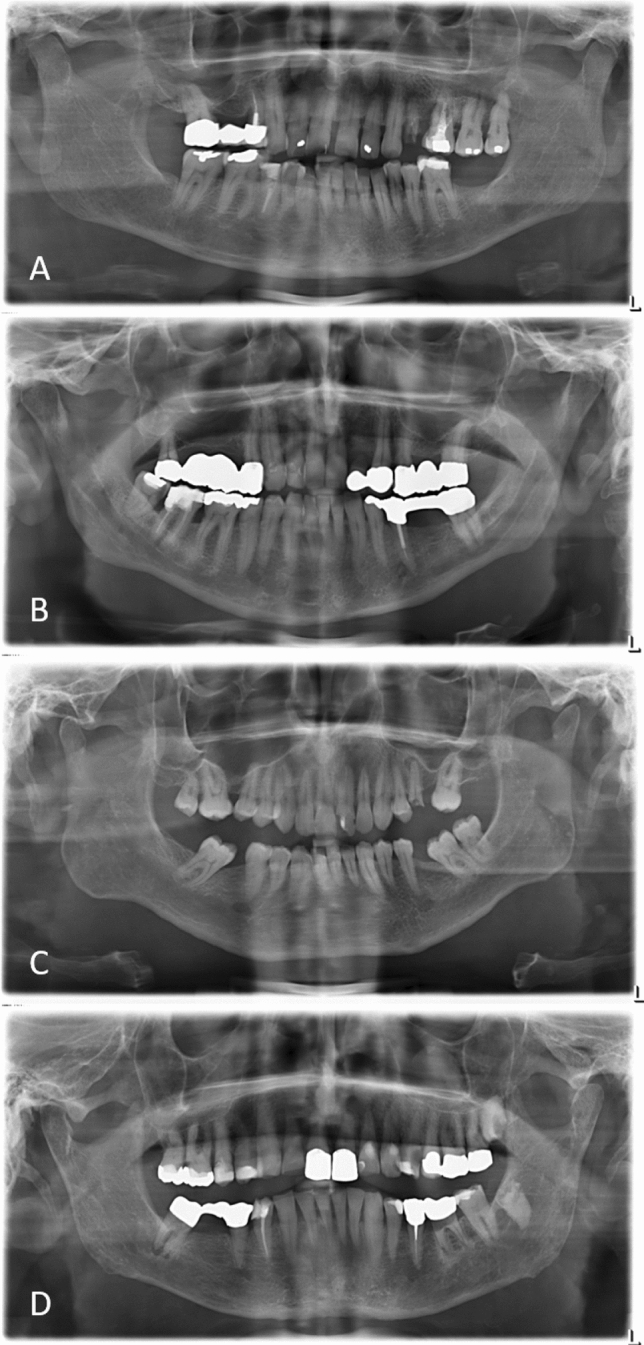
Exemplary demonstration of panoramic radiographs showing dense (A), heterogenous (B), sparse (C), and sparse with ground glass appearance (D) mandibular bone quality
